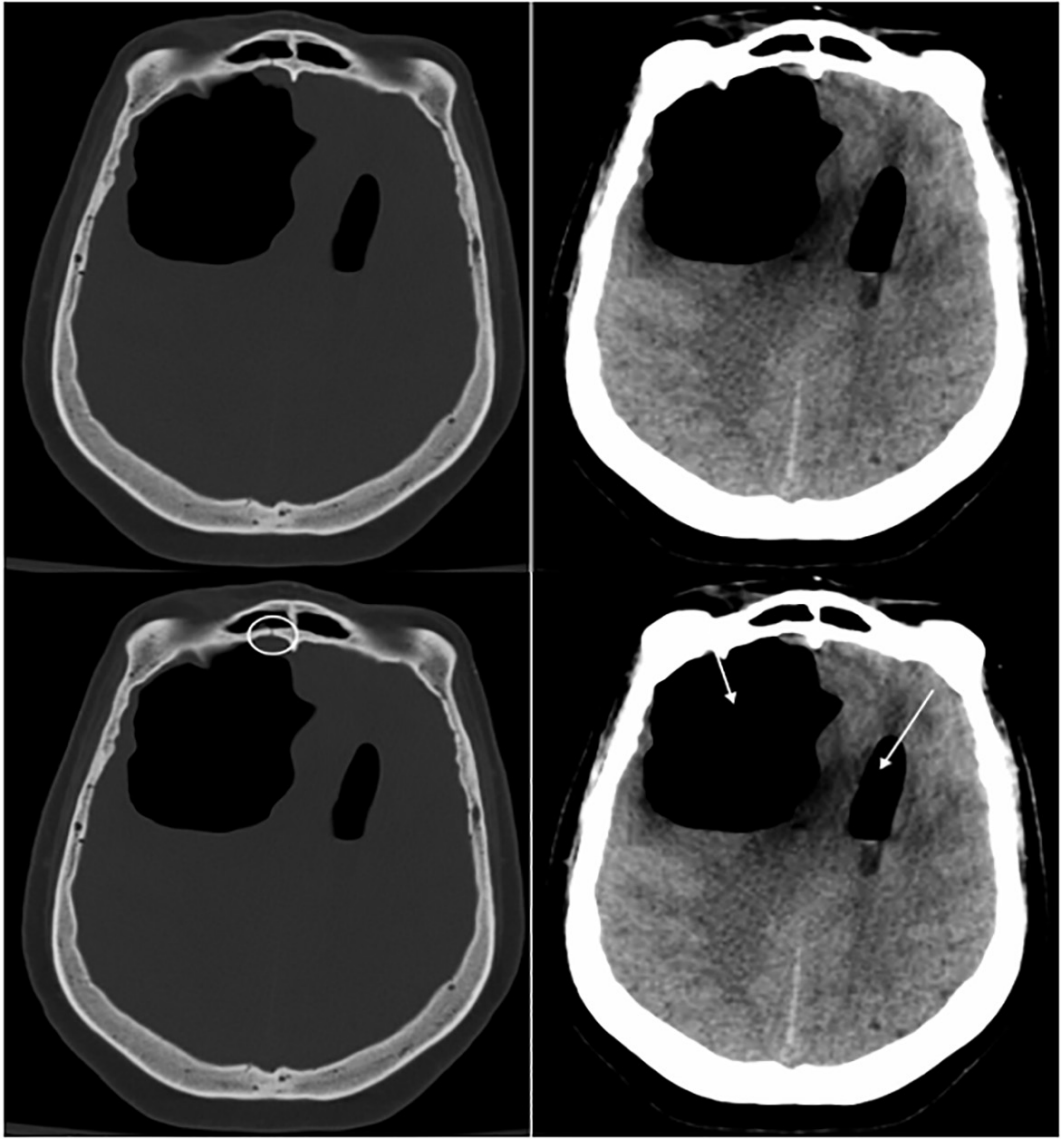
Computed tomography brain without contrast at presentation 21 days later.
